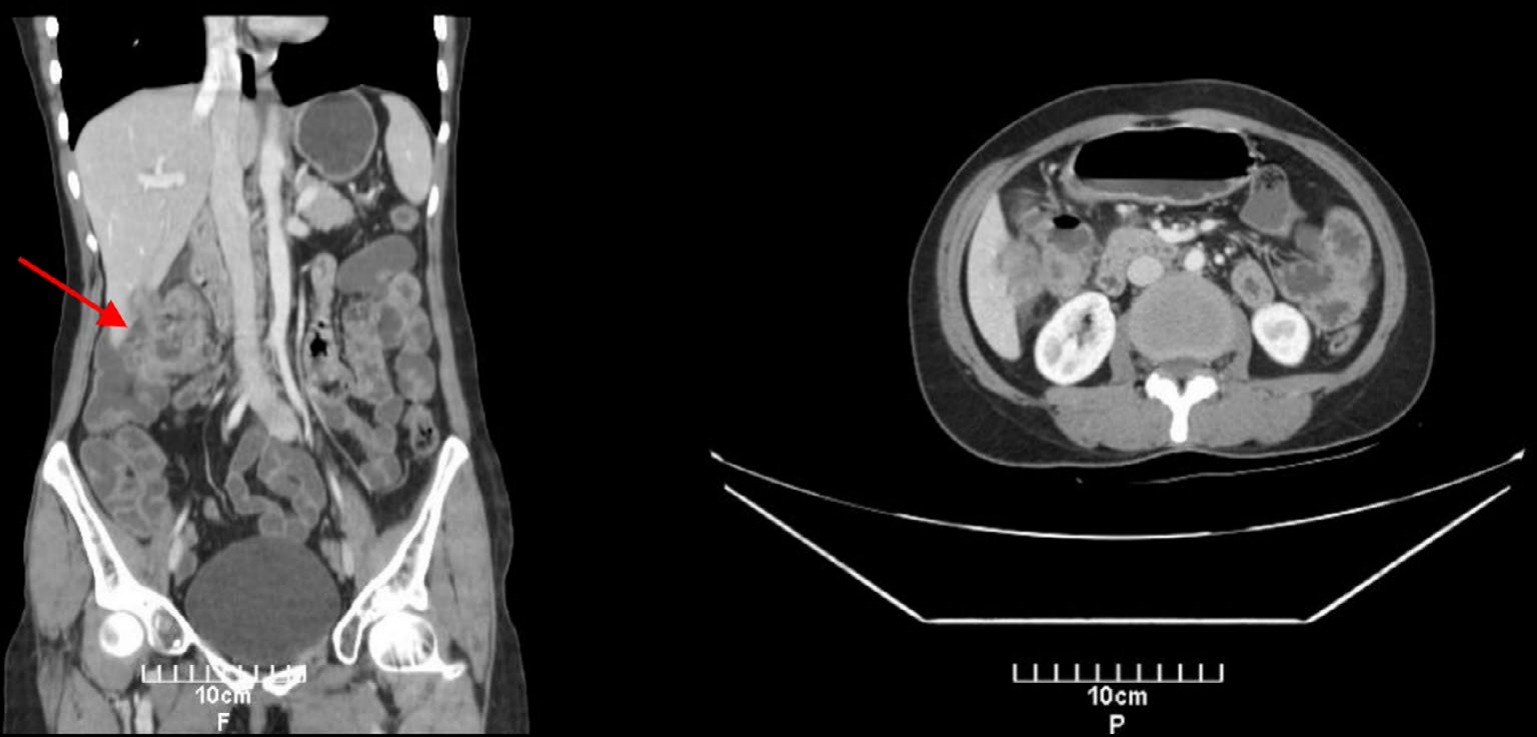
Contrast‐enhanced CT scan of the abdomen demonstrating a soft‐tissue mass in the ascending colon with circumferential wall thickening (arrow). There is local invasion to the liver and enlarged regional lymph nodes, suggestive of locally advanced colon cancer.
